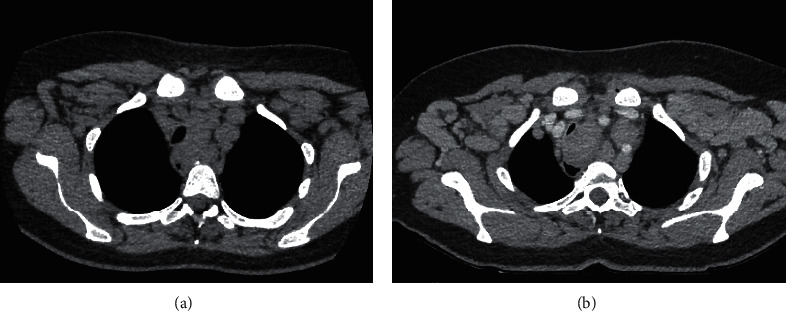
Computed tomography of the chest showing the rapidly expanding mediastinal lymphadenopathy. The trachea was compressed with the narrow diameter of <1 cm. (a) Pretreatment. (b) Day 14 following commencement of treatment.
